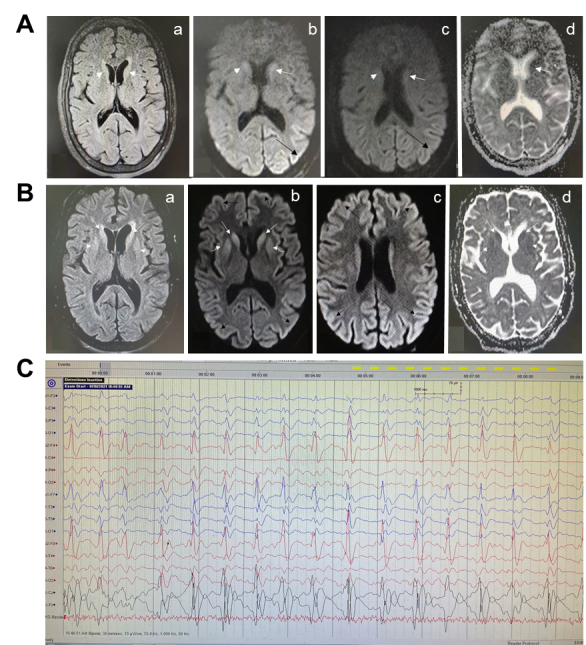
Specialized neurological investigations in the patient. (A) Magnetic resonance imaging of the brain performed after admission. Axial FLAIR (a), Diffusion b1000 (b, c) and ADC map (d) images show FLAIR hyperintensity in bilateral caudate head which shows diffusion restriction (white arrows). Further, there is patchy diffusion restriction in left sided gyri in posterior parietal and occipital lobes. (B) Magnetic resonance imaging of the brain performed after 16 days of initial imaging. Axial FLAIR (a), Diffusion b1000 (b, c) and ADC map (d) images of the same patient 16 days later shows increased degree of FLAIR hyperintensity and diffusion restriction in bilateral caudate (white arrow) as well as involvement of bilateral putamina (curved arrow). There is diffuse diffusion restriction in cerebral cortex in bilateral cerebral hemispheres (black arrow). Cortical ribboning is visible in diffusion weighted images. (C) Electroencephalogram of the patient. Electroencephalogram showing bilateral biphasic and triphasic sharp wave periodic discharges of around one Hz, with slowing of background activity.
